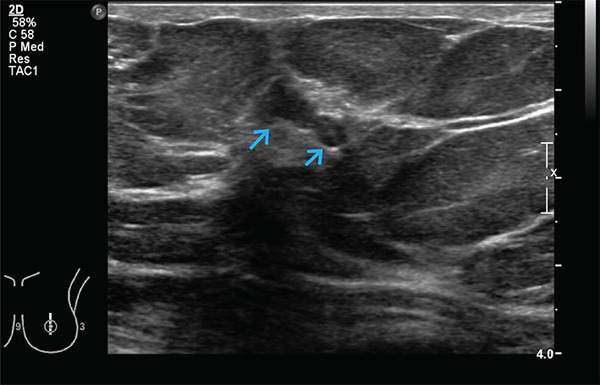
Targeted ultrasound of the subareolar left breast demonstrates a 12‐mm solid, oval, lobulated intraductal mass (blue arrows). BI‐RADS 4 (suspicious; biopsy recommended).
